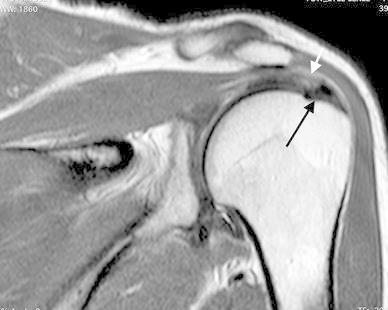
T1-weighted coronal oblique MRI shows a solid calcium deposit at the insertion of the SS (*black arrow*) with partial tear of the related tendon on the bursal side (*white arrow*)
